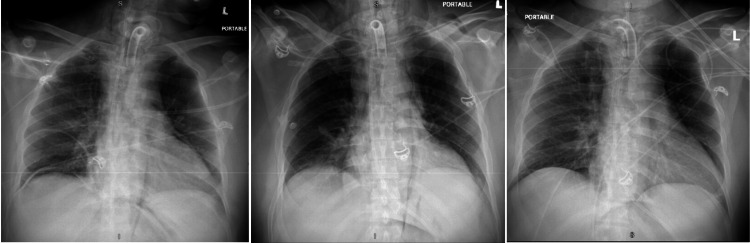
Resolution of subcutaneous emphysema, pneumomediastinum, and pneumoperitoneum seen on serial chest X-rays
